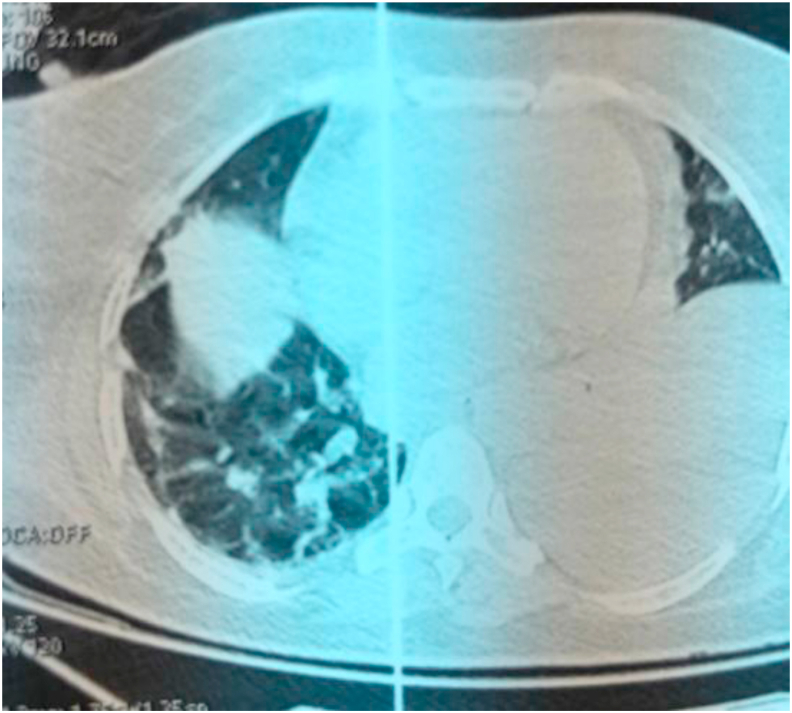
CT image showing multiple round and oval ground-glass opacities in both lungs, with a crazy-paving pattern in relation to COVID-19.
